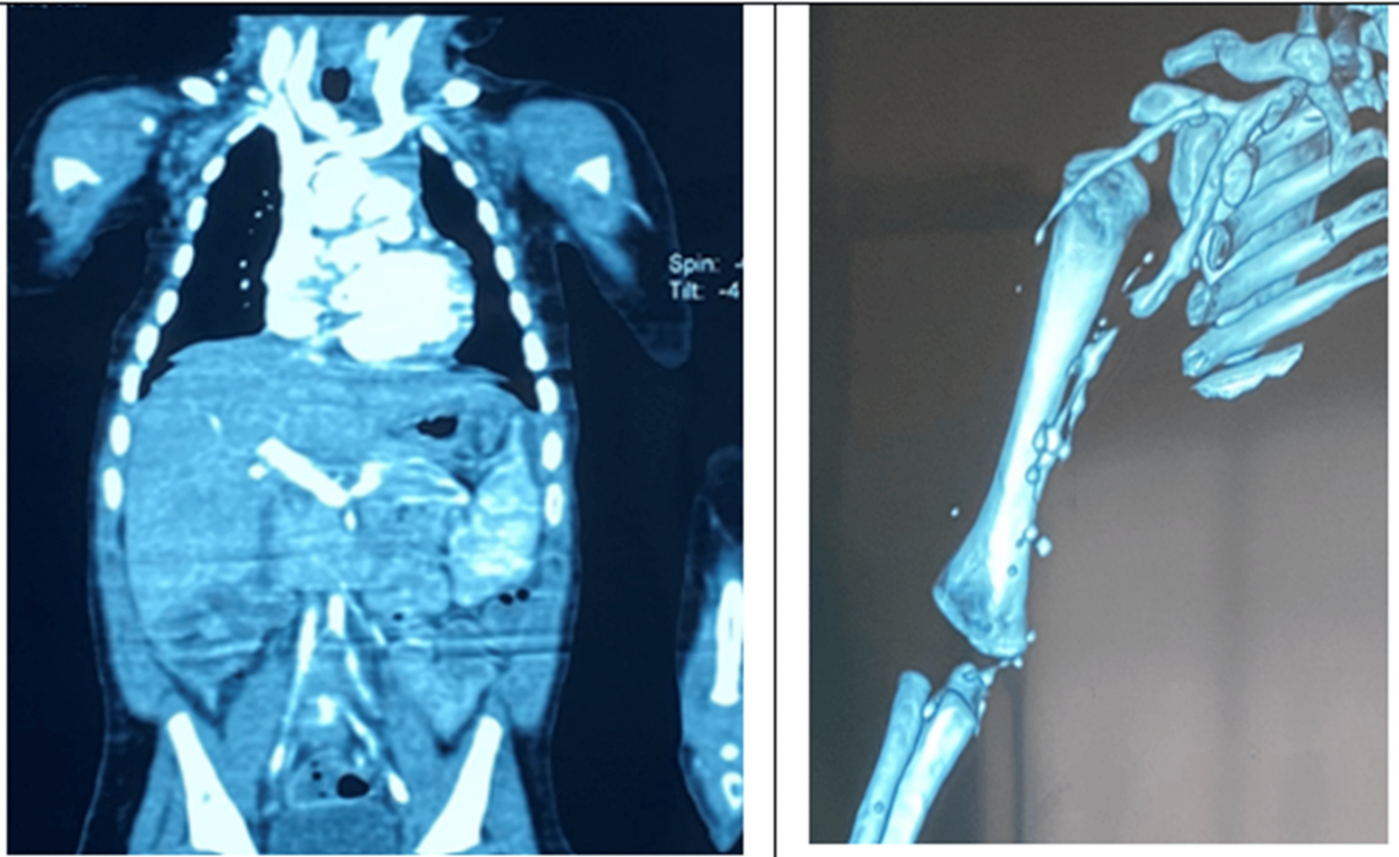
Computed tomography angiography demonstrating diffuse fusiform, saccular, and moniliform aneurysmal dilatations involving multiple medium-sized arteries
